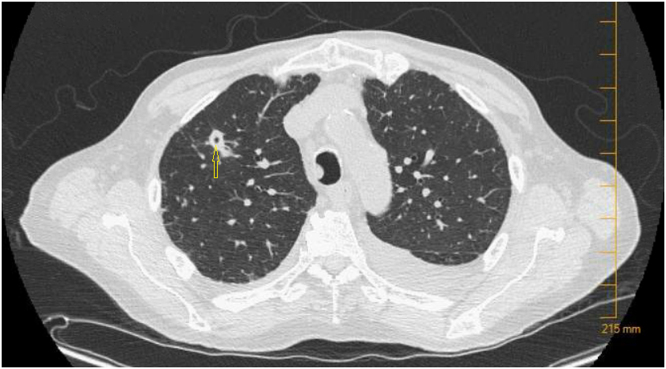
Computed tomography of the chest showed multiple, bilateral ill-defined nodular opacities. Yellow arrow pointing to a 1.3 × 0.9 cm nodule with central cavity in the right upper lobe.
